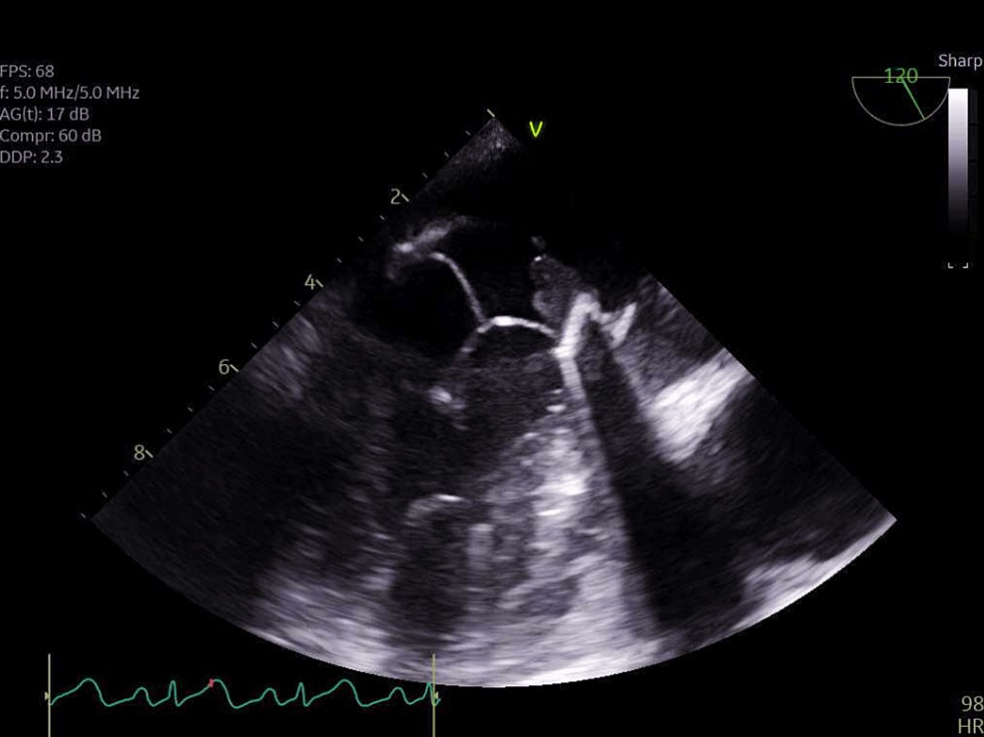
TEE image showing vegetations on the bioprosthetic mitral valve TEE, transesophageal echocardiogram
